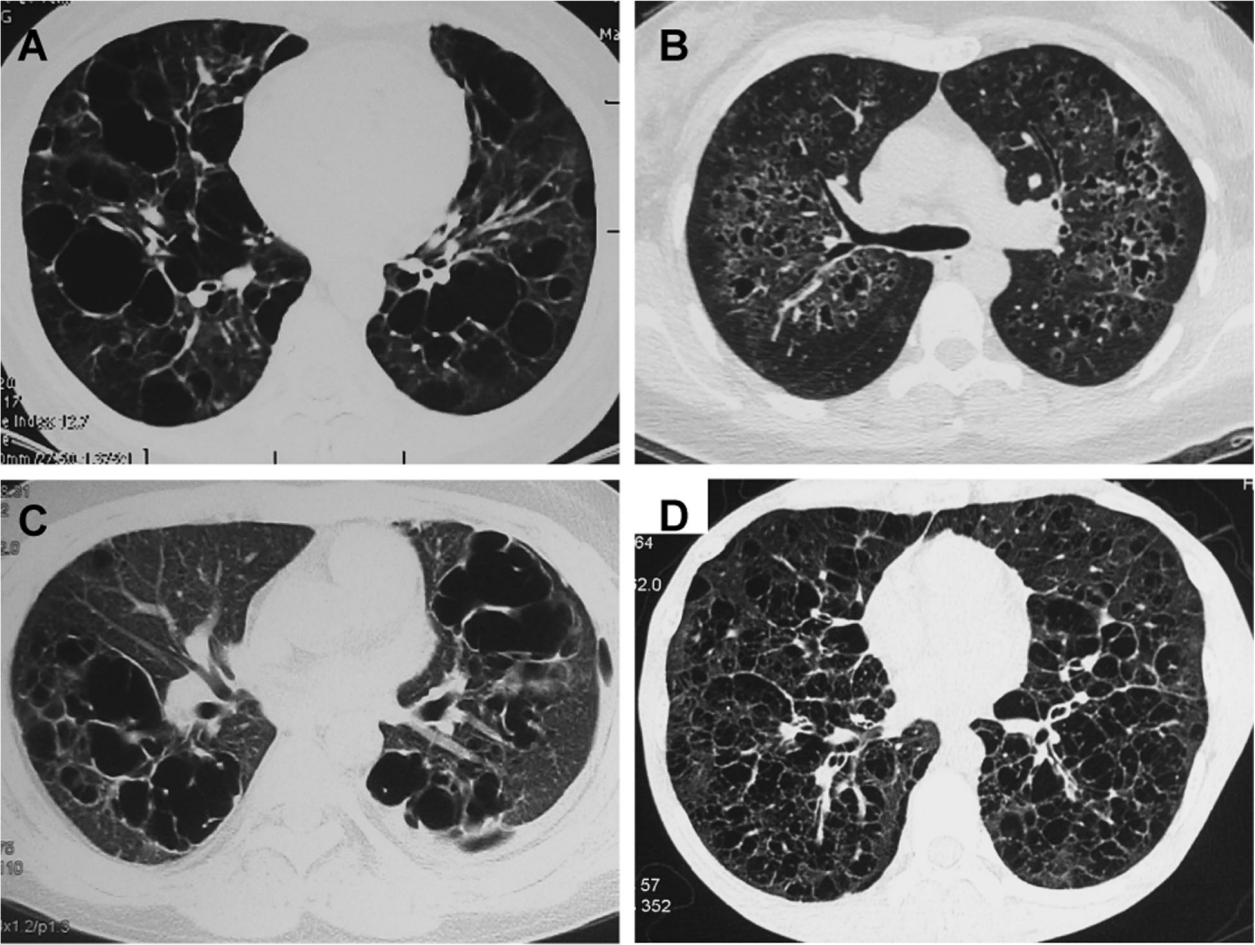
Thoracic CT findings of patients with non-BHD and non-LAM (NBNL) DCLDs. a CT images of patients with Sjogren’s syndrome; b CT images of patients with Pulmonary Langerhans Cell Histiocytosis; c CT images of patients with Langerhans Cell Histiocytosis; d CT images of patients with Castleman’s disease
